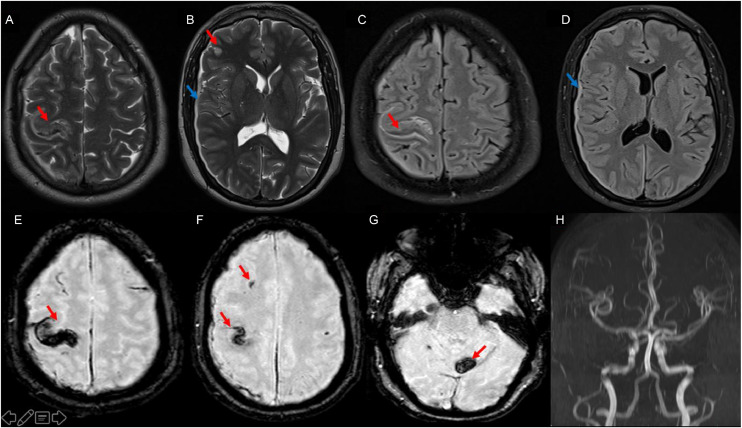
Axial MRI images: T2 (A,B), FLAIR (C,D), SWI (E–F-G), and arterial TOF (H) sequences show multiple hemorrhagic embolic lesions, hyperintense on T2/FLAIR and hypointense on SWI, with a left cerebellar hematoma (red arrows) and a right subdural hematoma (blue arrows).
